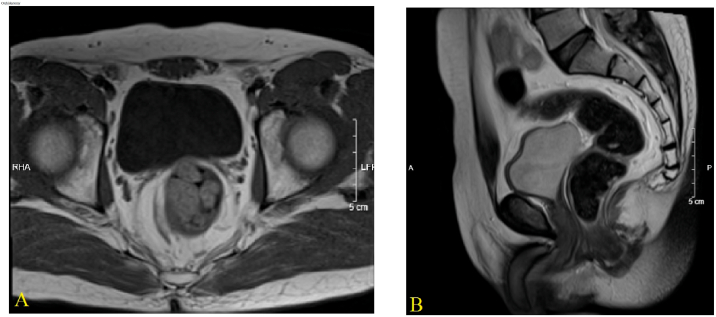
MRI shows no ovary, fallopian tube, uterus and superior part of the vagina, prostate and seminal vesicles. (A) MRI with cross section. (B) MRI with longitudinal section.
